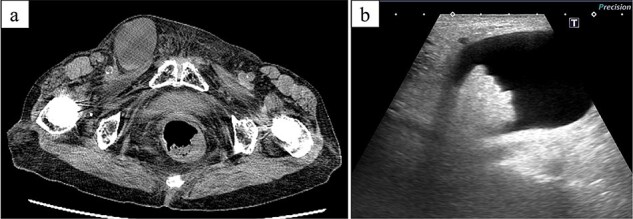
CT and ultrasonographic findings. (a) CT image showing a 4-cm right inguinal hydrocele. (b) Ultrasonography showing protruding fatty tissue within the hydrocele.
